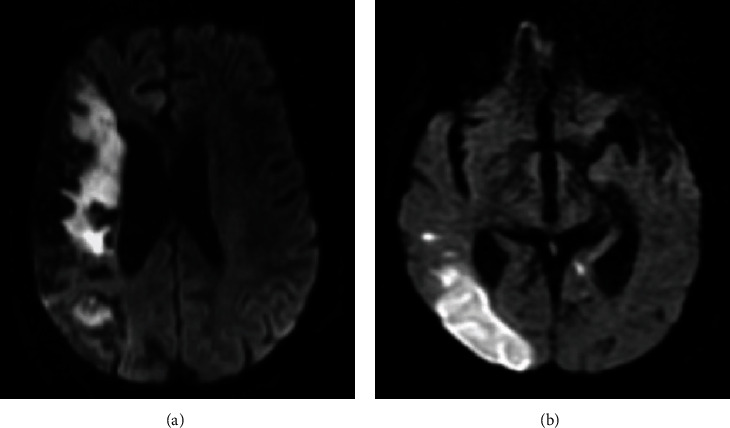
Samples of acute/subacute infarction in 2 different patients (a, b). (a) On DWI, right middle cerebral artery infarction was observed in a 69-year-old female patient affecting most of the right frontal and parietal lobes. (b) The diffusion restriction was observed in DWI due to large infarction in the posterior cerebral artery irrigation area in the right occipital lobe.
